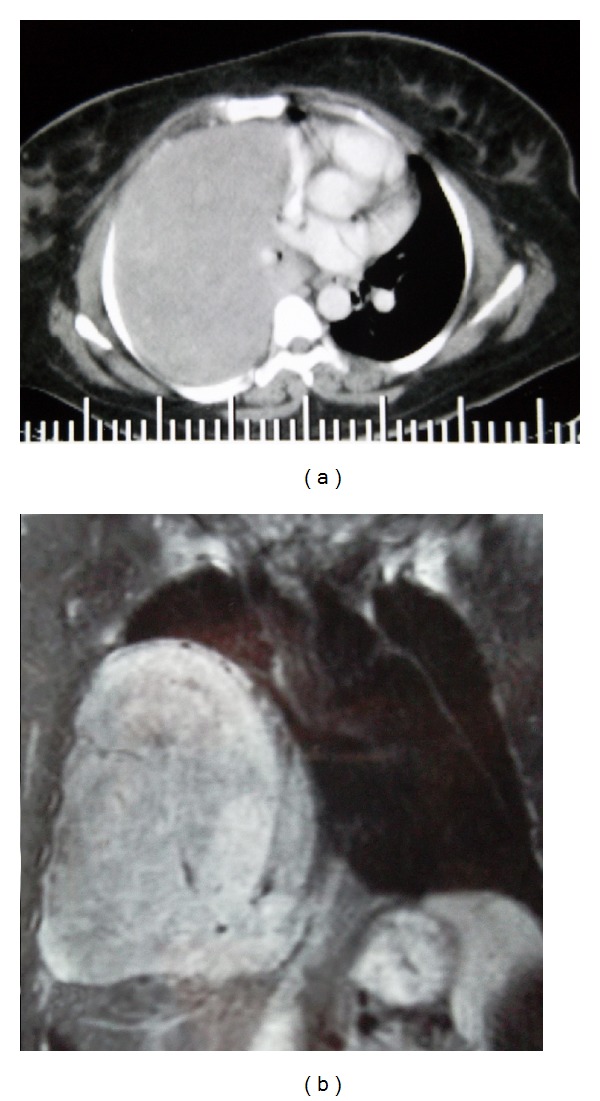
Giant mass can be seen that almost completely filled the right hemithorax on CT (a). Mediastinal shift was seen on MRI (b).
